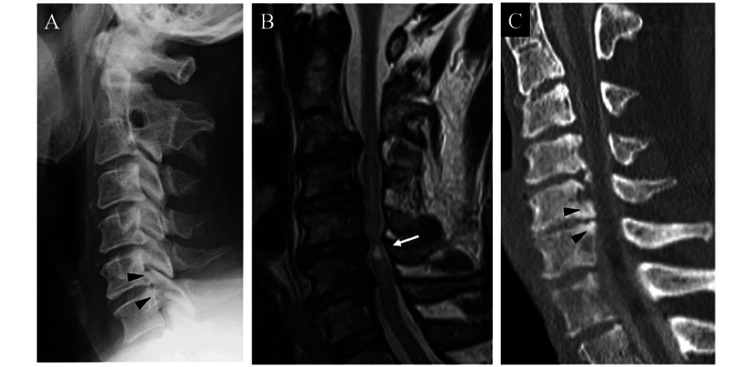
Imaging findings before surgery. A. Lateral X-ray image shows ossification of the posterior longitudinal ligament (arrowhead). B. MRI T2-weighted imaging shows a T2 high signal change at the C5/6 level (arrow). C. CT sagittal reconstruction image after myelogram shows ossification of the posterior longitudinal ligament (arrowhead).
